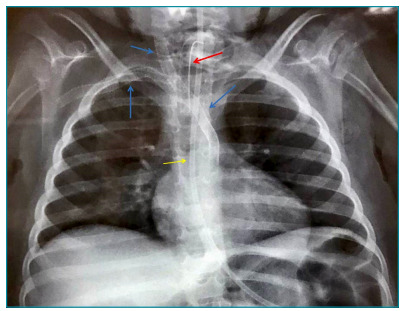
Thoracic radiography demonstrating the position of intra-arterial stents. Blue arrows: implanted polytetrafluoroethylene stents; red arrow: tracheostomy tube; yellow arrow: nasogastric tube.
